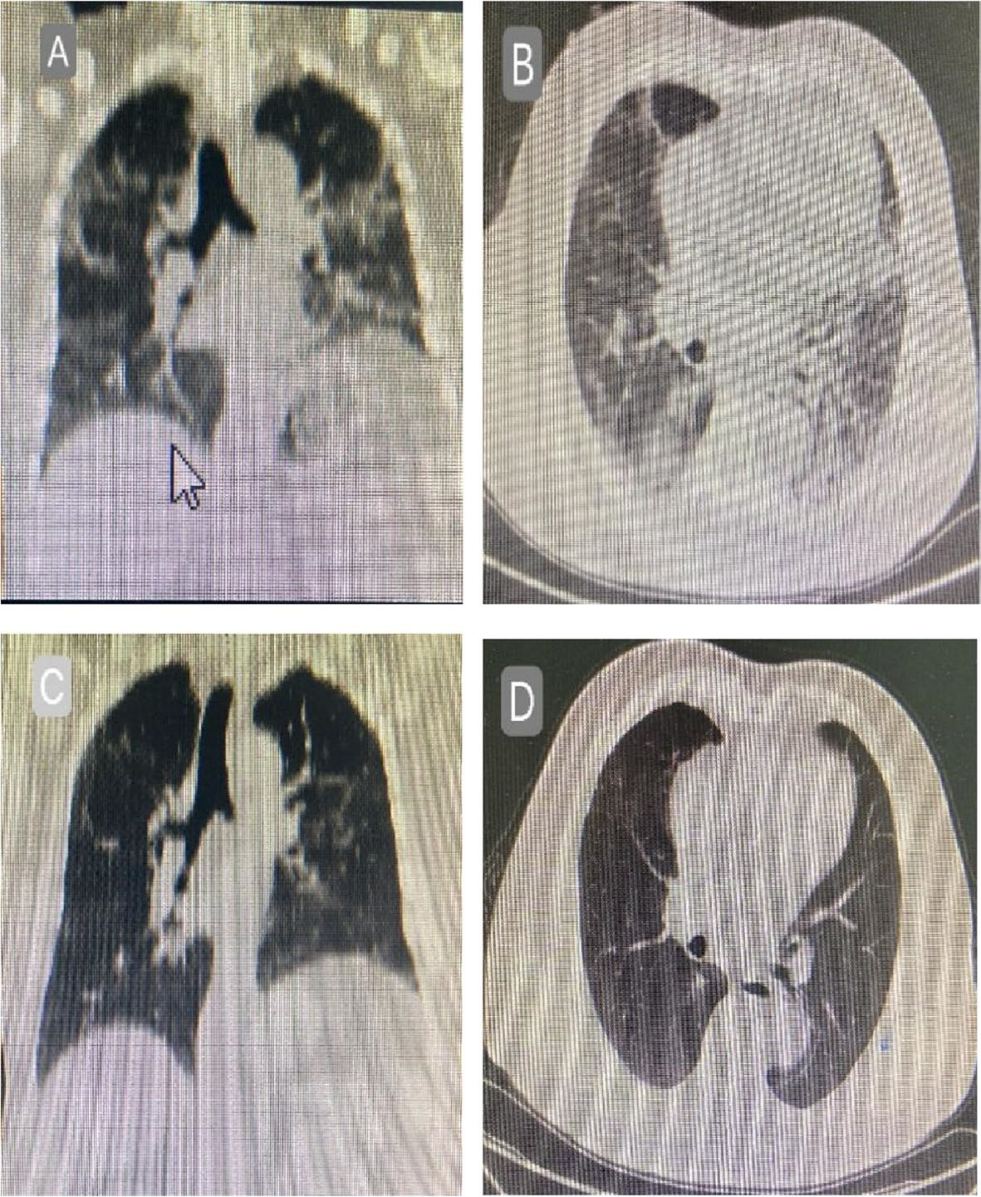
Coronal reconstruction and axial image from CT imaging of the chest acquired when discharged in a 73-year-old man (A and B) shows a radiological pattern with predominant peribronchial and perilobular dense consolidation mild traction bronchiectasis of the airways. Follow-up CT imaging of the chest acquired after 1 month of oral prednisolone (C and D) shows residual ground glass and a little subpleural consolidation. As shown in the image below)
